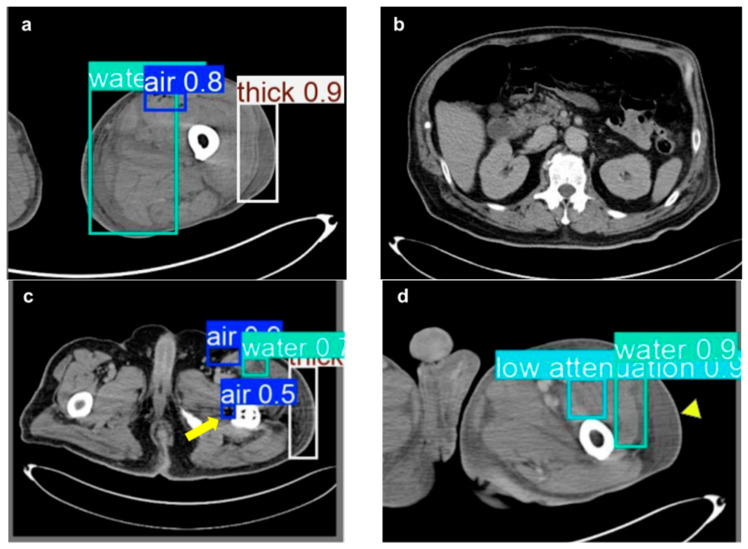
Detection examples visualizing the model’s performance on test set CT images. (a) Multiple features correctly detected in the NSTI image. (b) No NSTI features found in healthy images. (c) Implant shadow misclassified (yellow arrow). (d) Missed fascia edematous change (yellow arrowhead).
